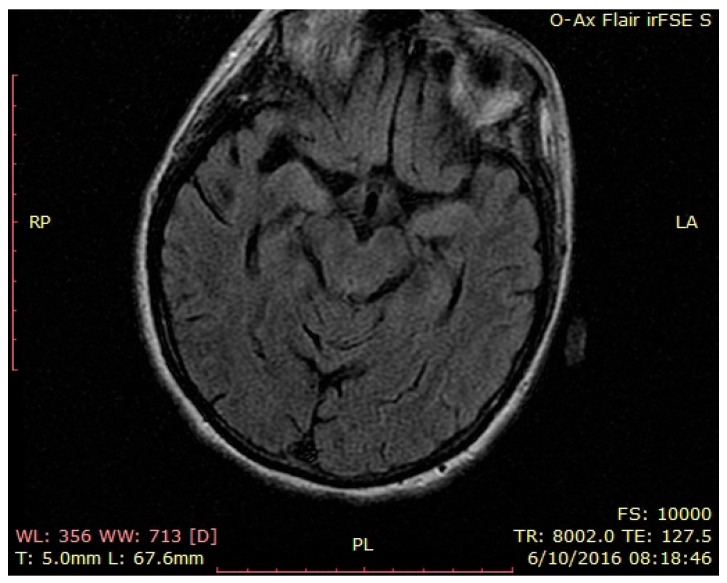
Second brain MRI, FLAIR images, axial temporal image.
